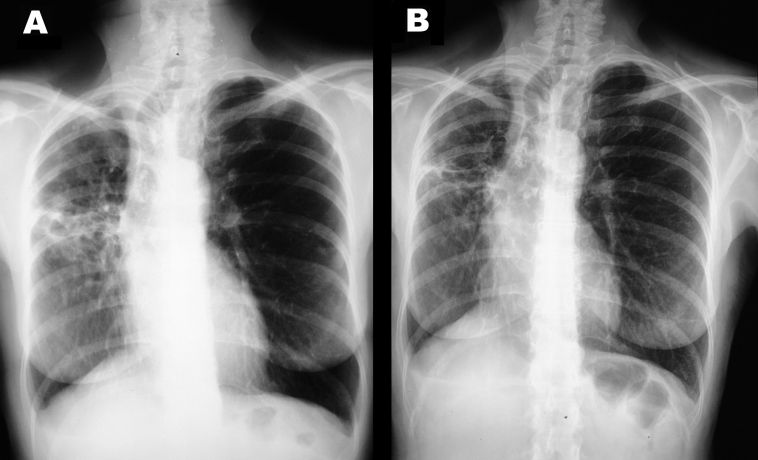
Chest x-ray showing nodular lesions and lung cavitation before (A) and after (B) treatment.
